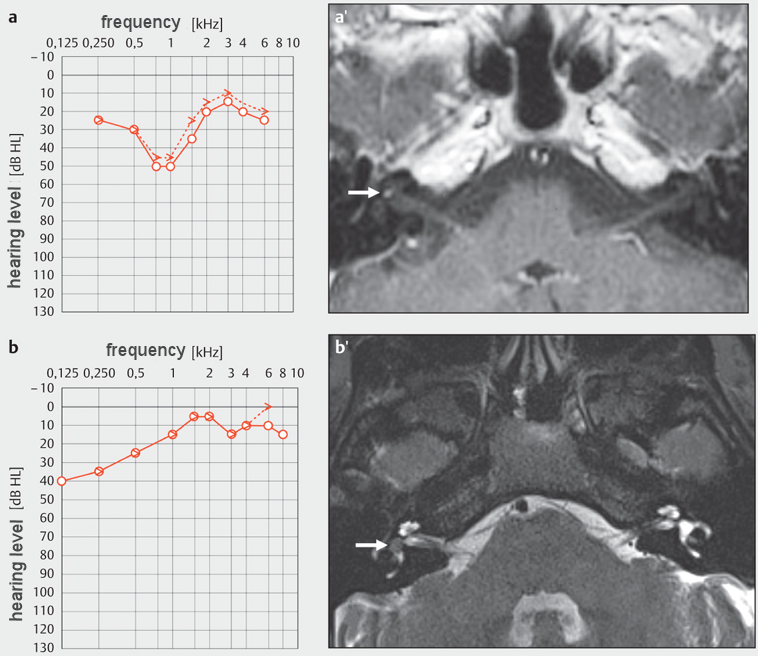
Intralabyrinthine schwannomas (a intracochlear; b intravestibular) with symptoms of sudden hearing loss: a, a’: Because of the symptoms of sudden hearing loss with mild to moderate medio-cochlear hearing loss, a MRI (axial, T1-w, contrast agent) had been performed in 2005. It showed a very small contrast enhancing mass in the right cochlea (arrow). In the course of 10 years, the tumor showed a progressive growth until it filled the whole cochlea. It was removed via subtotal cochleoectomy with partial reconstruction of the cochlea and insertion of a CI electrode dummy. The functions of the semicircular canals were preserved [53]. b, b’: Intravestibular schwannoma in the vestibule (arrow, T2-w, axial) with Menière’s disease like complaints and mild acute hearing loss in the lower frequency range. After increasing vertigo, the tumor was resected via labyrinthectomy and in the same session hearing rehabilitation was performed with cochlear implantation. CM: contrast medium; w: weighted. (b’: Department of Radiology, University Medicine Halle, courtesy of Prof. Dr. S. Kösling).
